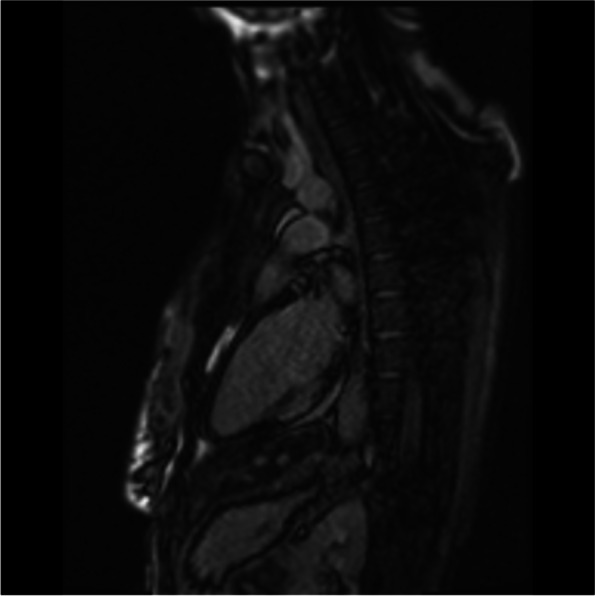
Cardiac MRI, inverse recovery LGE sequence, long axis view, showing transmural late gadolinium enhancement of the mid inferior segment in an ischemic distribution pattern
